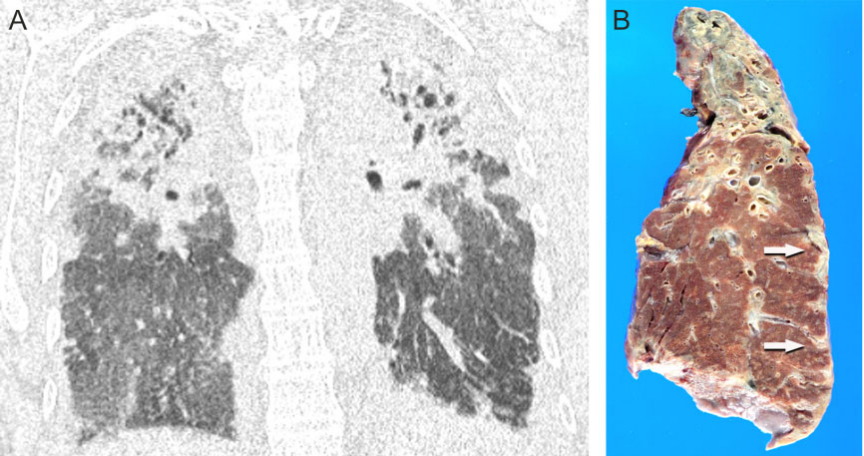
(A) Coronal reformatted computed tomography scan showing consolidative density effacing both upper lobes with pleural thickening noted extending to the lower zones. Cystic bronchiectasis is present. (B) Gross photograph of a coronal section of the left lung showing collapse and fibrosis of the entire upper lobe. Pleural fibrosis with nodular accentuation is noted in the lateral aspect (arrows).
